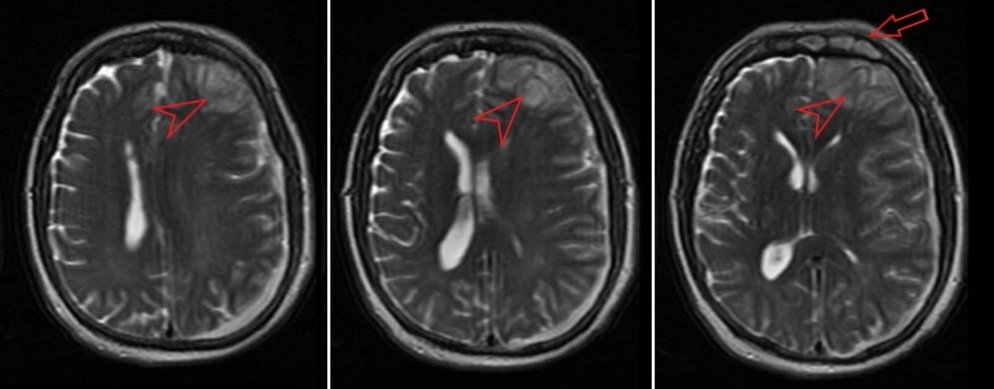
T2 weighted magnetic resonance imaging (MRI) showing complex left-sided acute subdural fluid collection with left frontal abscess (arrowhead), and sinusitis (arrow).
